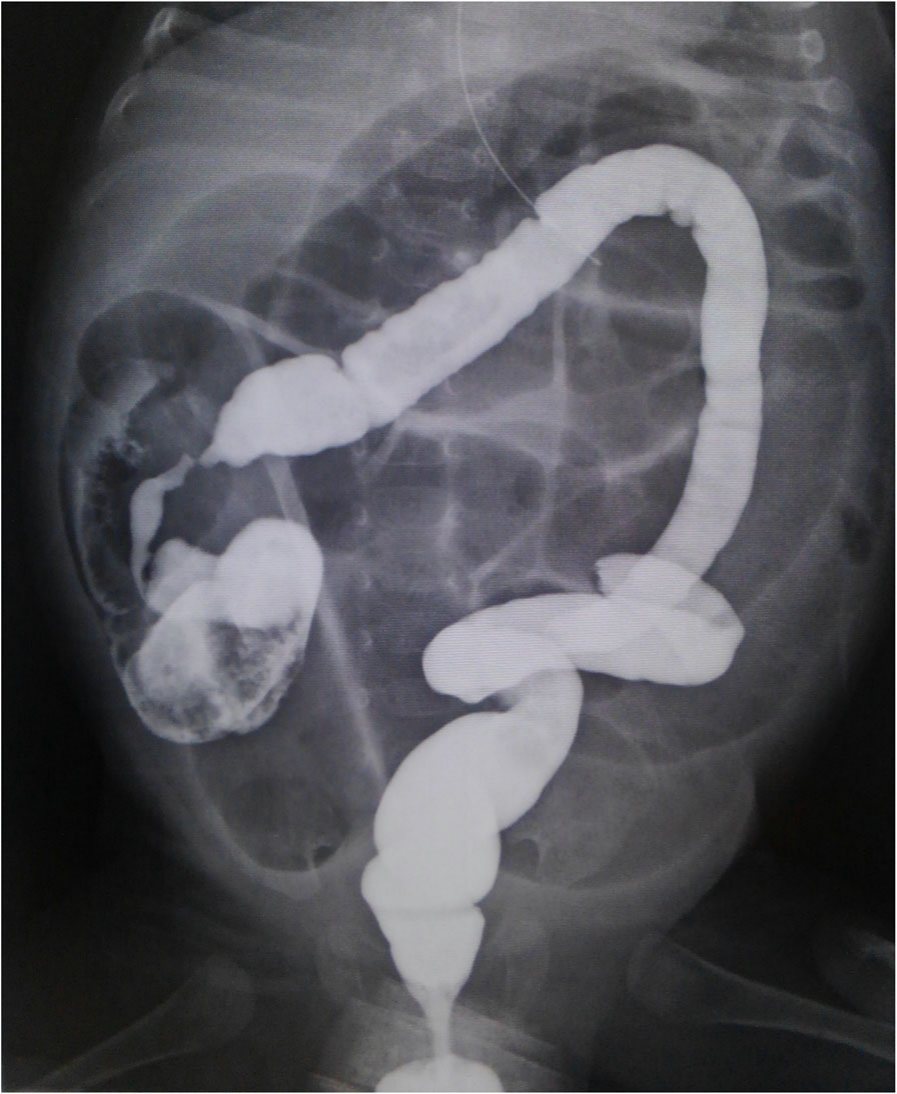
Contrast X-ray of the colon on day 50. Beak signs of ascending to the transverse colon suggested colon stenosis
